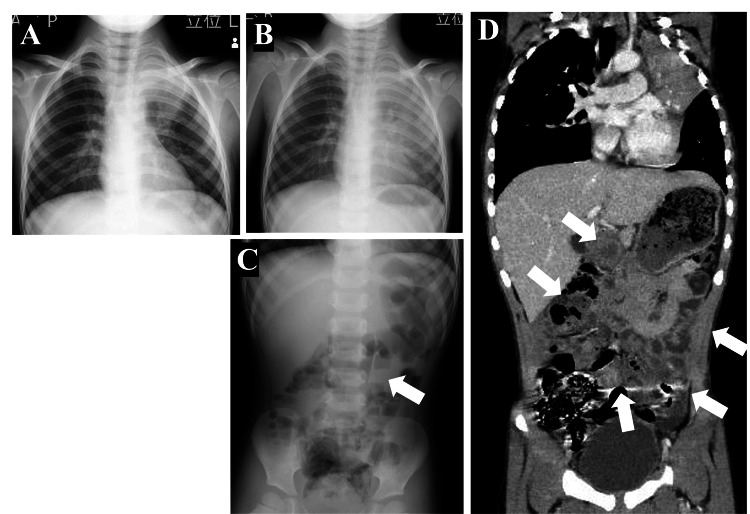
Radiologic findings throughout the clinical course. A: Initial chest radiograph (day five of illness) showing localized consolidation in the left upper lung field, consistent with pneumonia. B: Chest radiograph obtained on admission (day eight) demonstrates progression to diffuse consolidation in the entire left lung, particularly in the upper lobe, with obscuration of the left heart border (silhouette sign). C: Upright abdominal radiograph on admission indicates a step-ladder pattern of air-fluid levels in the small bowel, suggesting the presence of localized ileus (white arrow). D: Contrast-enhanced abdominal CT scan on day 11 shows extensive fluid accumulation in the small bowel (white arrows), reflecting impaired intestinal motility. No evidence of mechanical obstruction was identified.
